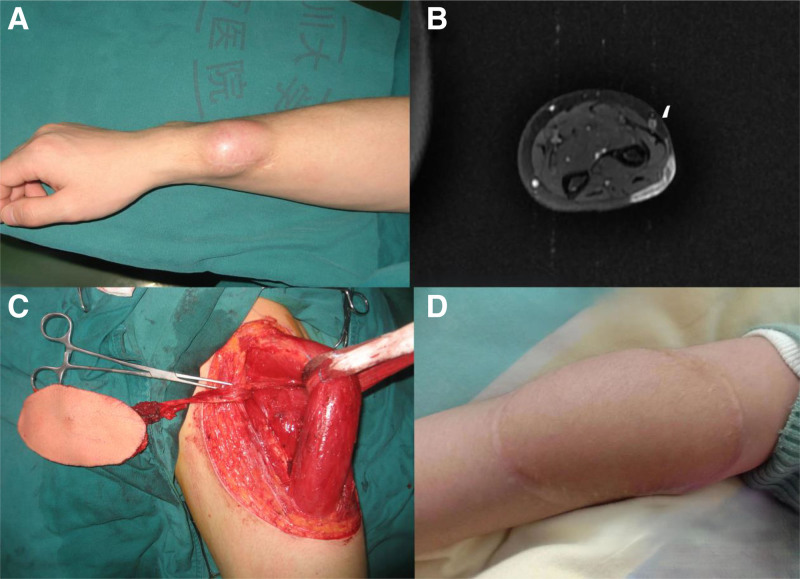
Clinical presentation and MRI findings of case 4. (A) DFSP lesion located in the forearm; (B) Magnetic resonance imaging before surgery; (C) free anterolateral thigh flap; (D) post-operative picture.
